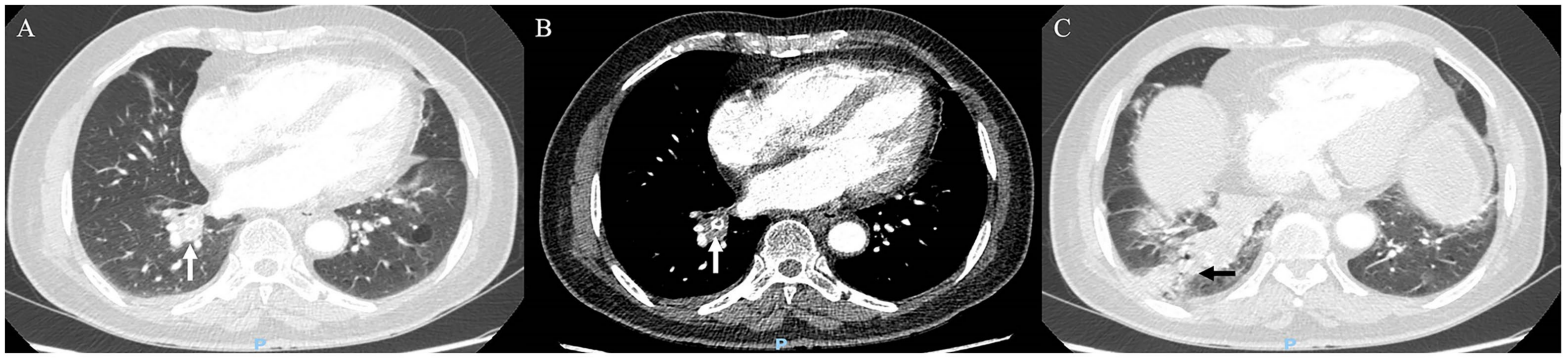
Serial chest computed tomography (CT) imaging of Case 2. (A) Axial CT scan (lung window) showed a hyperdense annular focus (white arrowhead) at the opening of the posterior basal segment of the right lower lobe. (B) Mediastinal-window setting accentuated the hyperdense annular focus (white arrowhead), strongly suggesting an aspirated foreign body. (C) Axial CT scan (lung window) showed associated post-obstructive atelectasis of the right lower lobe posterior basal segments, with dilated, mucus-filled bronchi (black arrow).
